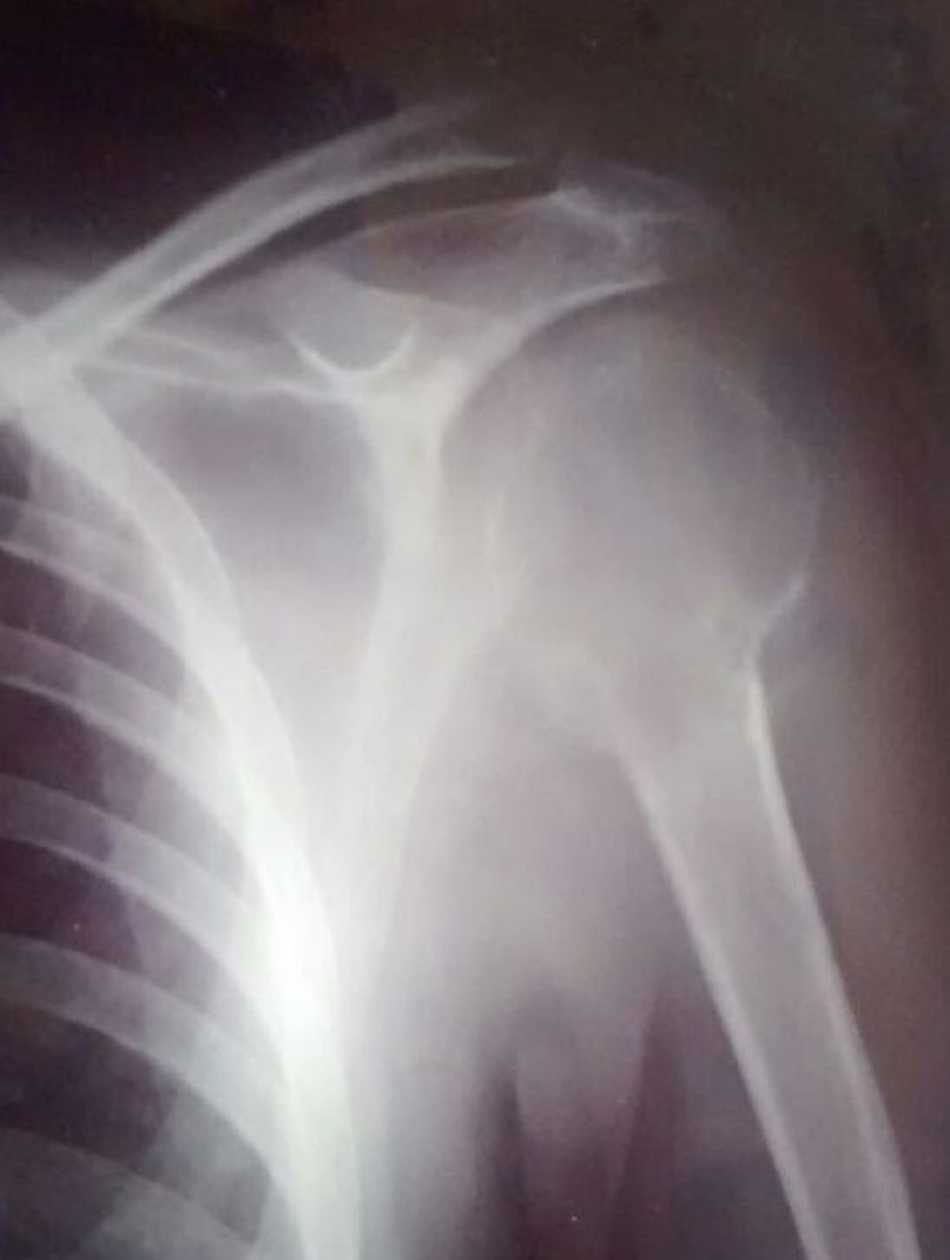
Anteroposterior radiograph of the left shoulder (Case #1) showing a large lytic lesion involving the whole humeral head and proximal humerus with indistinct cortices and uniform radiolucency.
